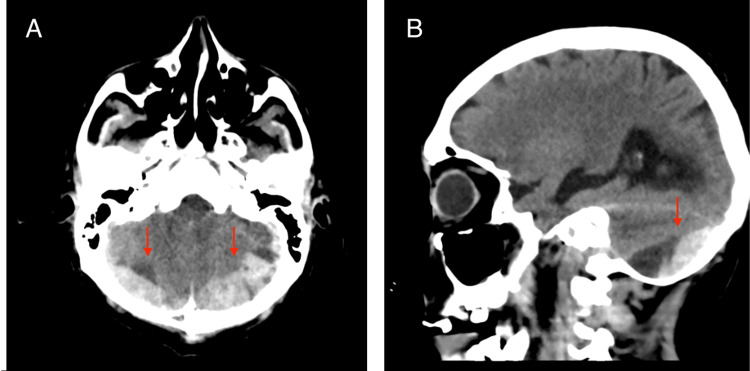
Brain CT scan (A) Axial brain CT image showing bilateral acute infratentorial subdural hematomas (arrows) with tentorial extension, resulting in significant posterior fossa mass effect. (B) Sagittal brain CT image demonstrating compression of the fourth ventricle and brainstem displacement, consistent with obstructive hydrocephalus. These findings correlated with acute neurological deterioration and prompted emergent neurosurgical decompression.
